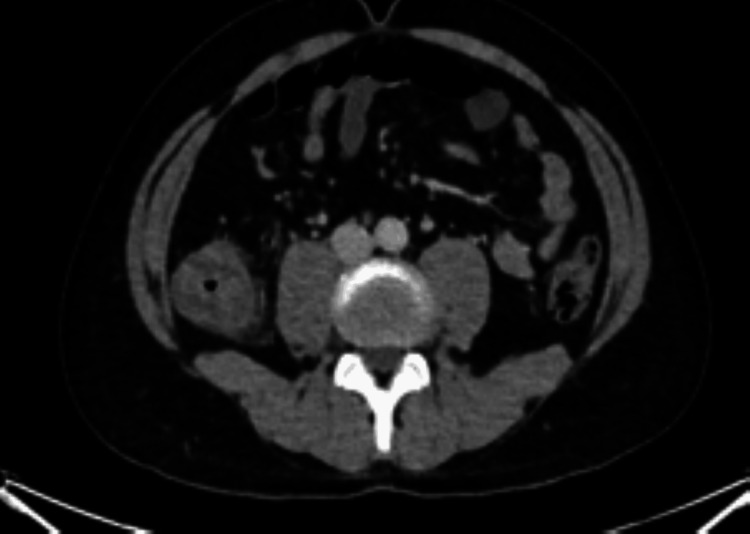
CT demonstrated a thickened ascending colon after the onset of obstructive symptoms.
